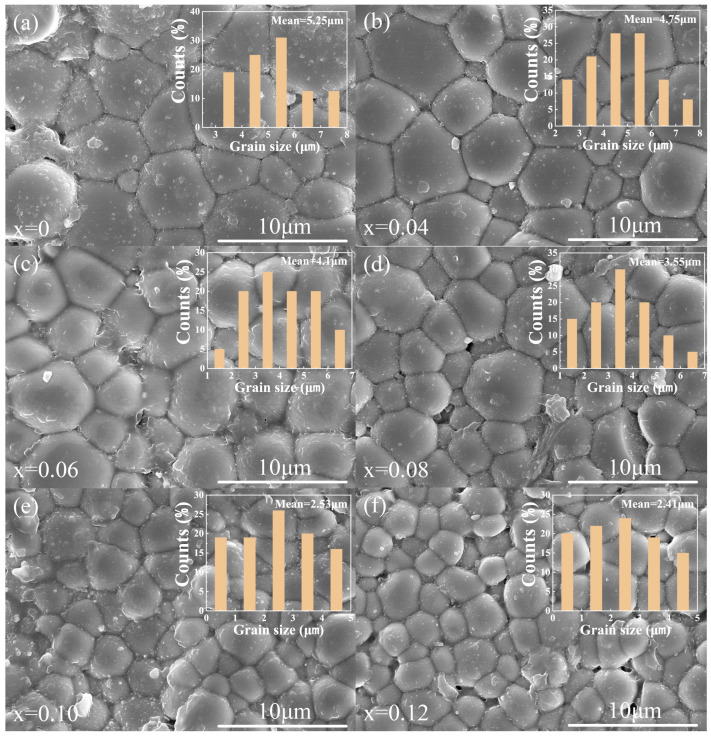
(a–f) The surface SEM images of various BSNT-*x*CT ceramics.
